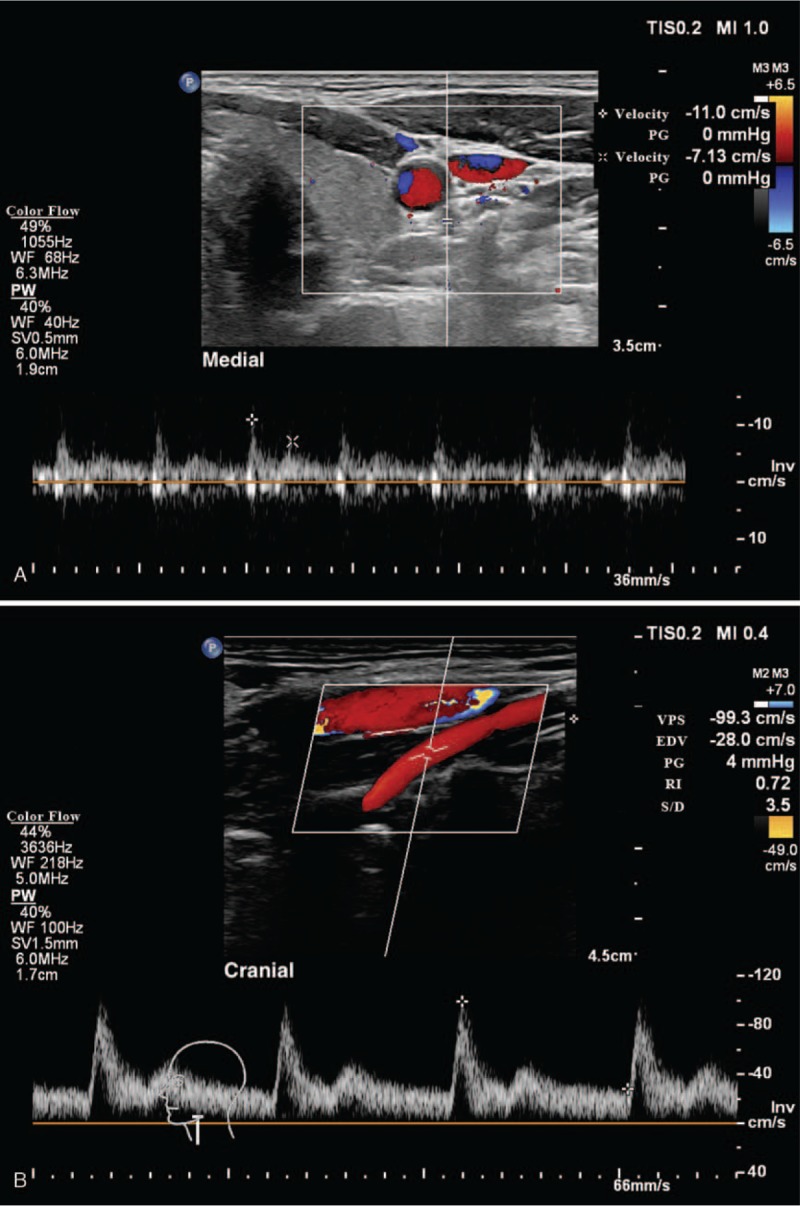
The color Doppler ultrasound image of the vertebral artery. (a) The M-mode ultrasound image of the vertebral artery at C6 level; (b) The color Doppler ultrasound image showed the left vertebral artery entered the transverse foramen at C5.
